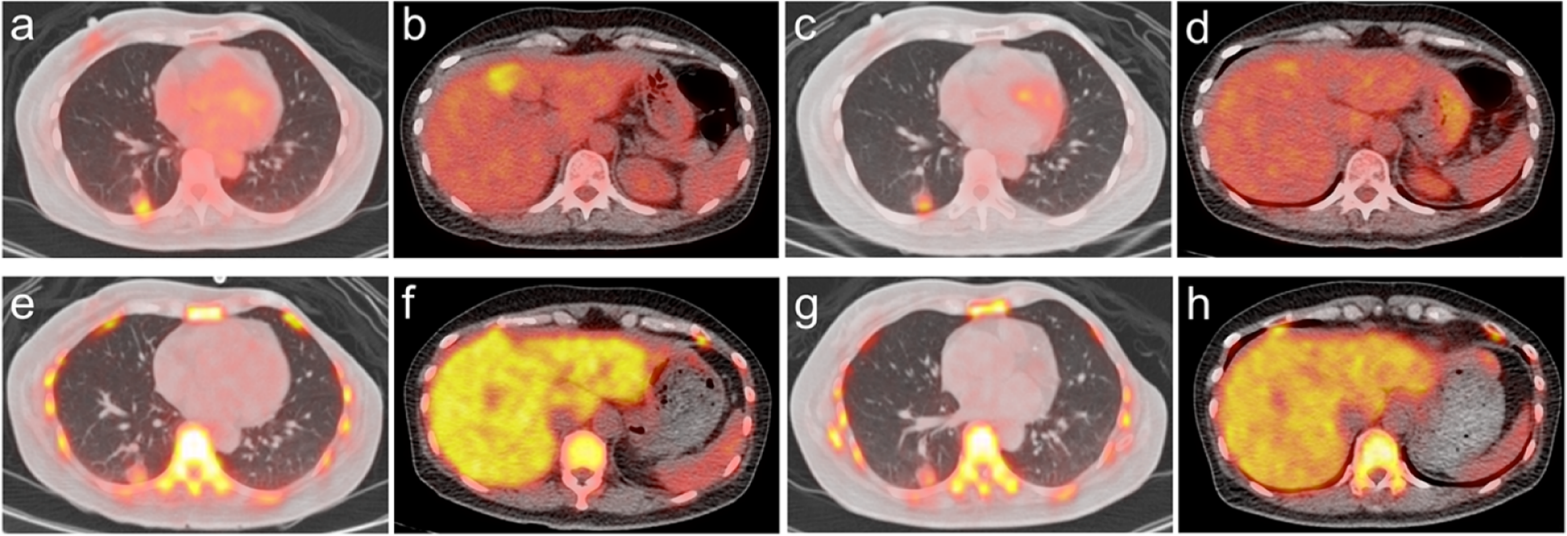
A case of a 51-year-old female presenting with metastatic breast cancer at the right lower lung and liver. (A, B) baseline ^18^F-FDG-PET, (C, D) post-2c ^18^F-FDG-PET, (E, F) baseline ^18^F-FLT-PET, and (G, H) post-2c ^18^F-FLT-PET
